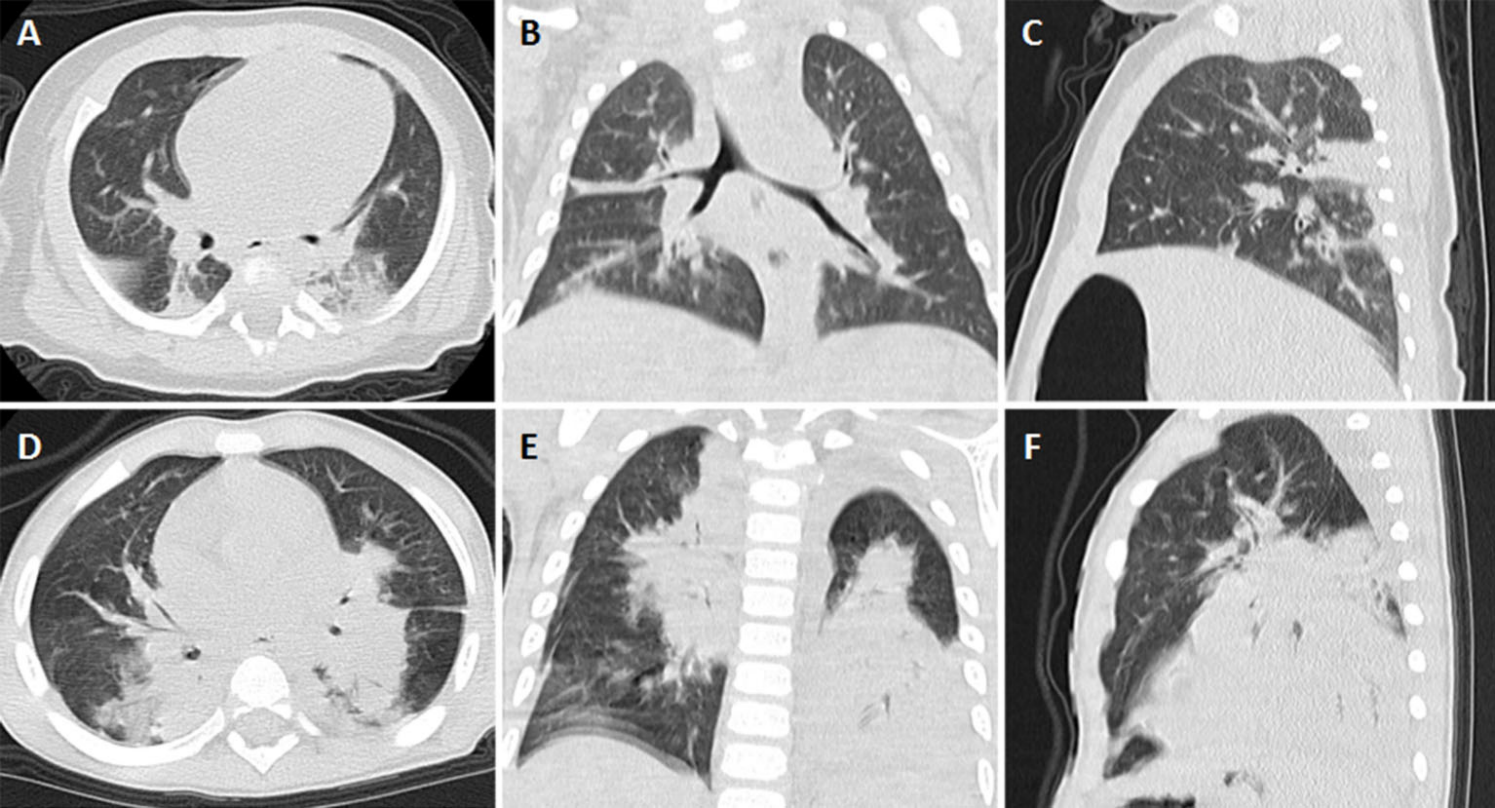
Imaging features of mild and severe human adenoviruses (HAdV) pneumonia patients. A-C, High-resolution CT scan of the chest at different sections on the day of admission revealing diffuse infiltration and few areas of consolidation in right upper lobe and bilateral lower lobe pneumonia in a three-year-old child with mild HAdV pneumonia; D-F, High-resolution CT scan of the chest at different sections on the day of admission showing large bilateral consolidation in each lobe and small bilateral pleural effusion in a three-year-old child with severe HAdV pneumonia.
